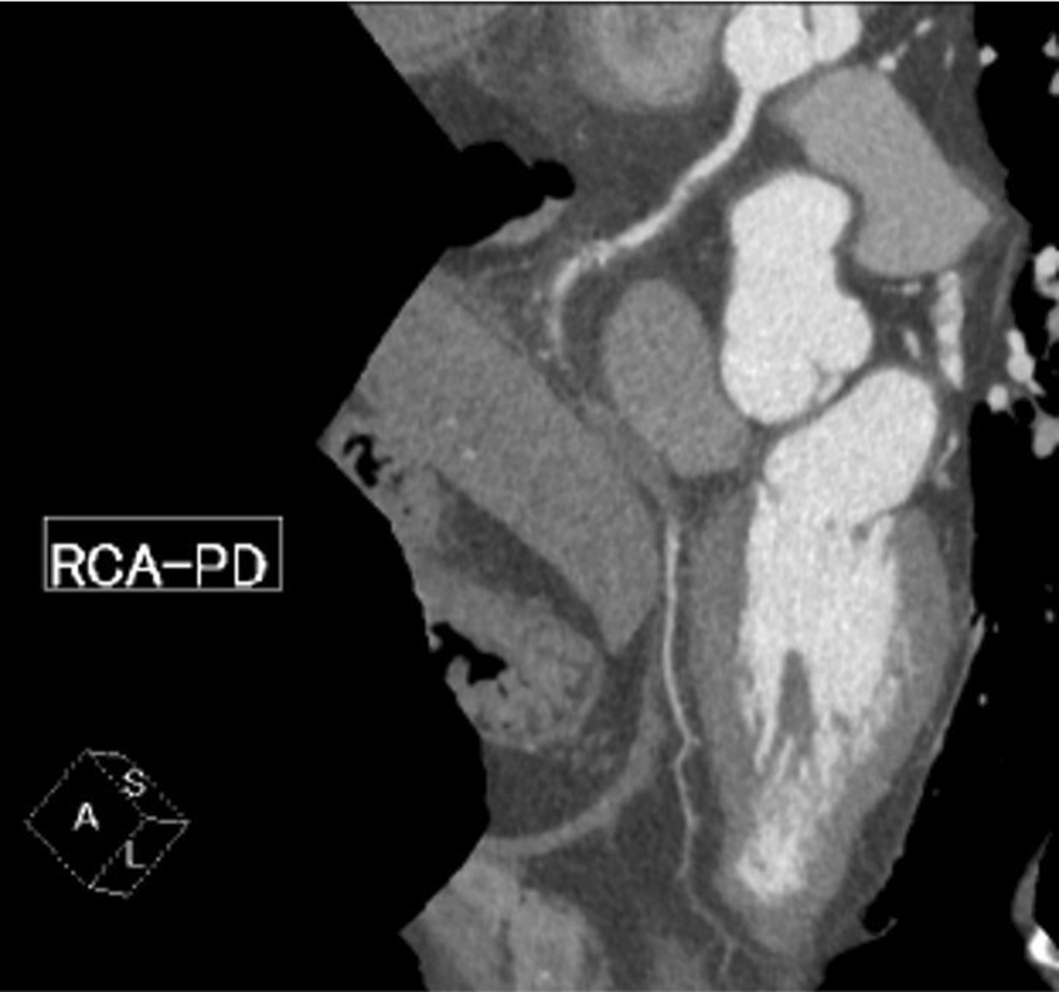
Coronary computed tomography angiography. Follow‐up coronary computed tomographic angiography reveals a residual thrombus in the right coronary artery.
